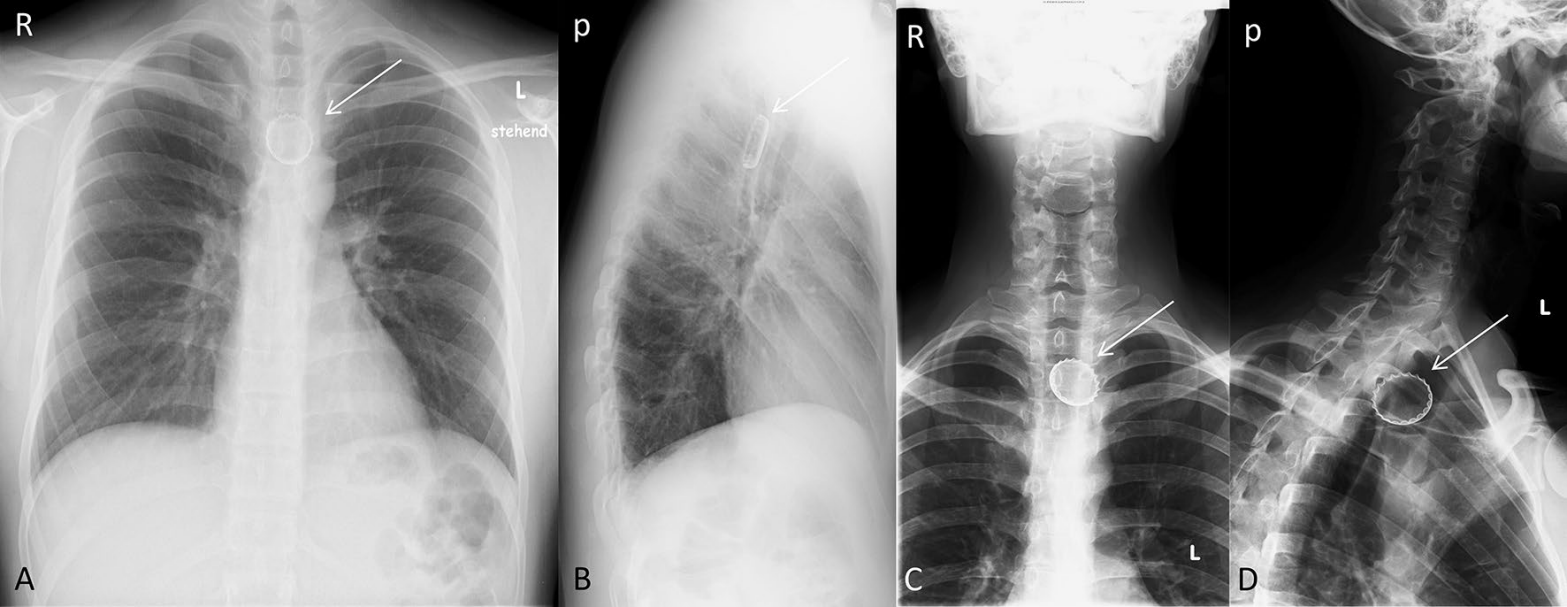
Middle esophageal narrowing. Point where the esophagus passes by the aorta and the left main bronchus. It lies at the level near of thoracic vertebrae IV. This is about 10 cm caudal to the upper narrowness. A, B: Posterior–anterior X-ray of the thorax (A) and left-sided lateral X-ray. Bottlecap near thoracic vertebra IV/V (arrow). C, D: Anterior–posterior (C) and left-sided lateral X-ray of the cervical spine (D). Bottlecap near thoracic vertebra II/III (arrow). *R* right; *L* Left; *a* anterior; *p* posterior
